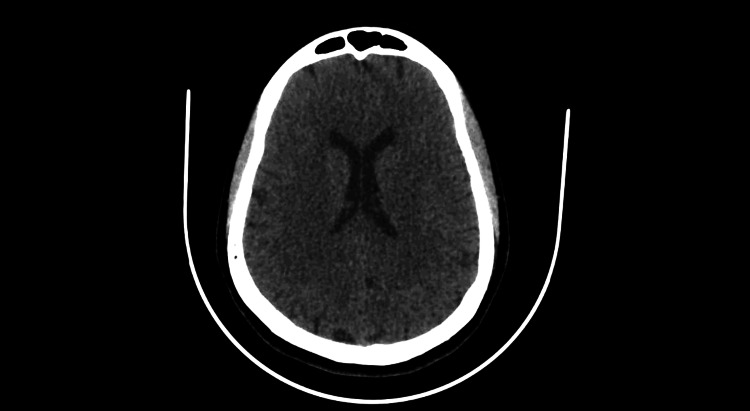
CT Brain showing no abnormal changes.
